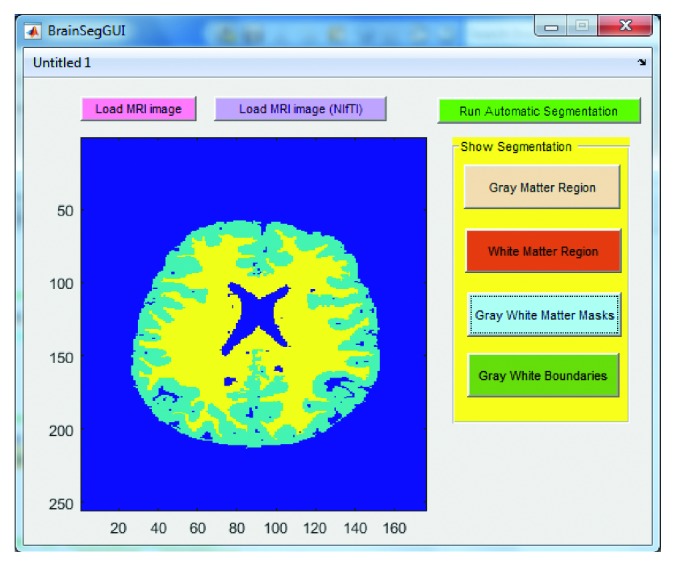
Screenshot of the graphical user interface (GUI) designed and developed in this work for automatic brain MRI image processing. Step shown here is to show the gray and white matter masks upon the click of the “Gray White Matter Masks” button.
